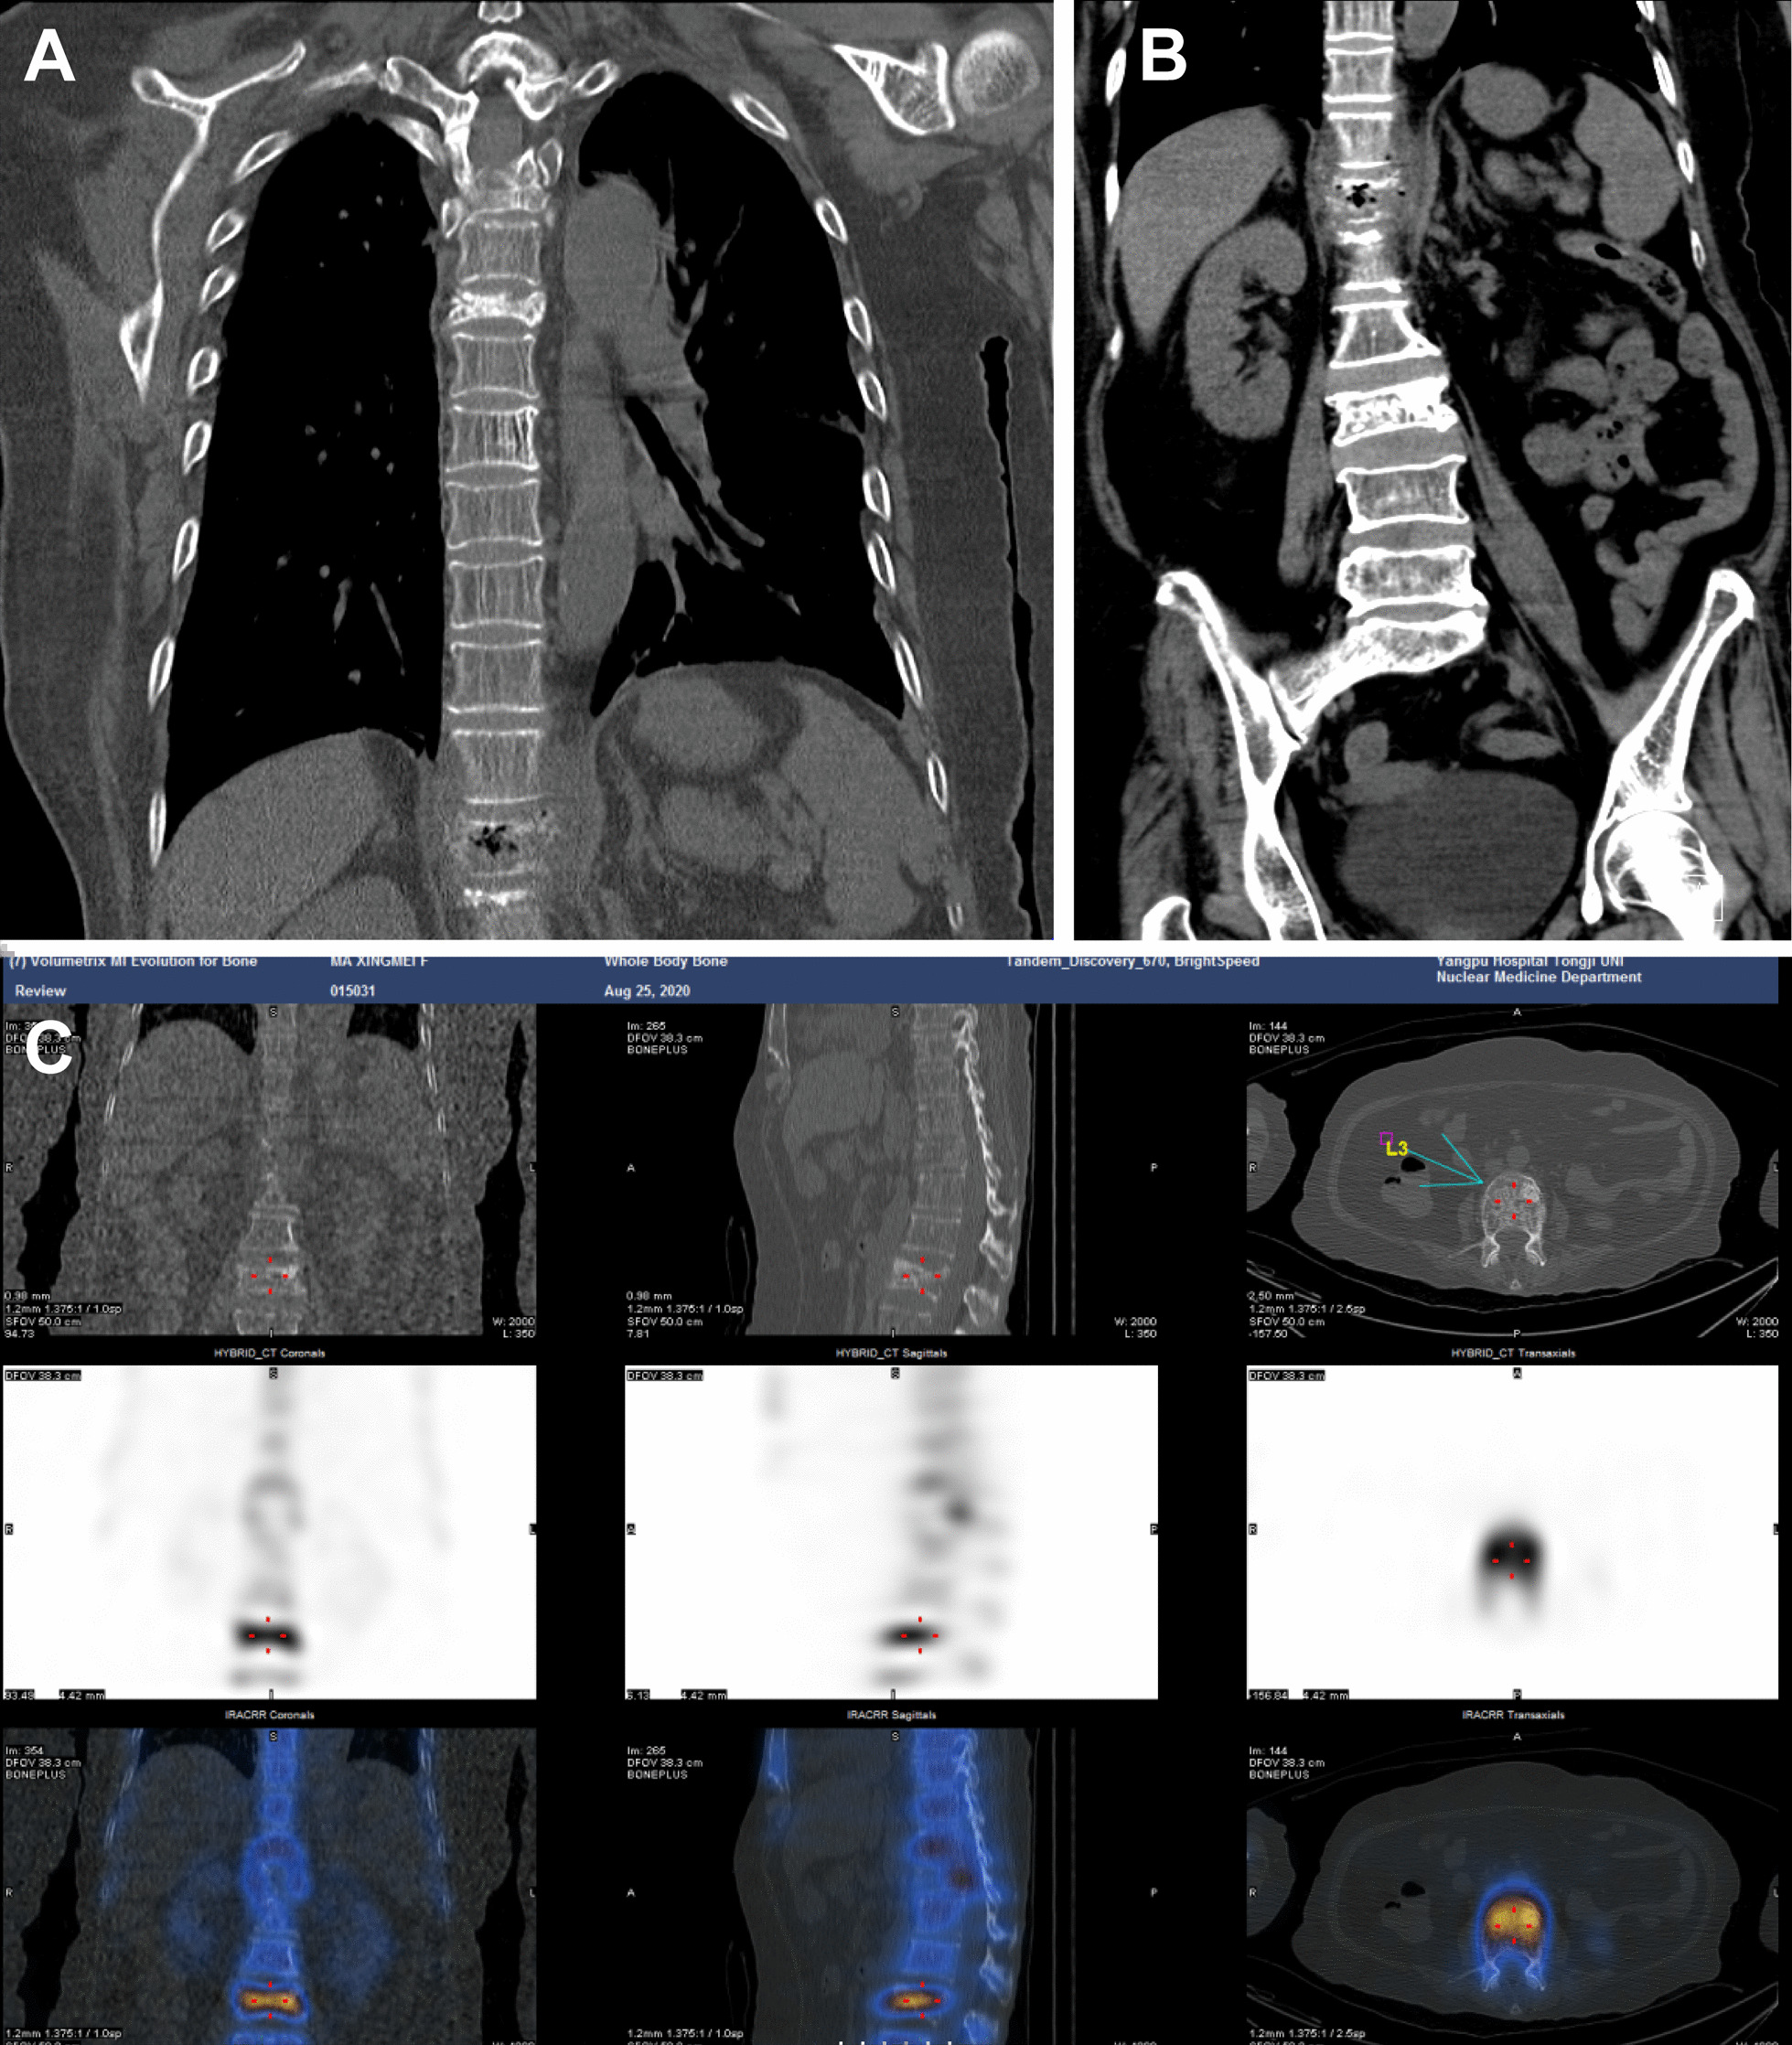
Thoracolumbar imaging findings. A Chest CT on August 18, 2020. B Abdominal CT on August 18, 2020. C Radionuclide scan on August 25, 2020
